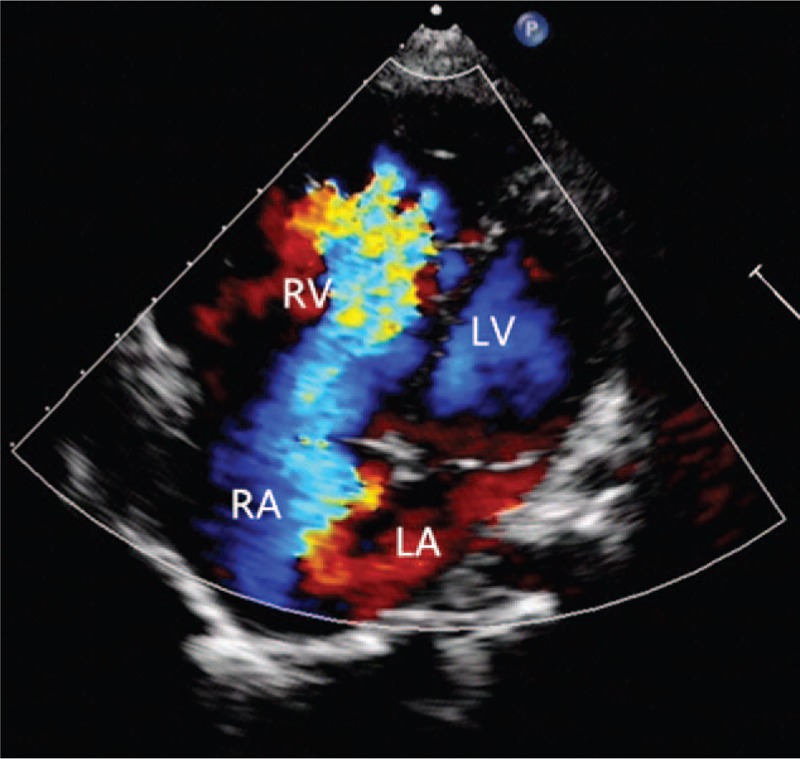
Echocardiographic image in the apical 4-chamber view demonstrated systolic severe tricuspid regurgitation.
